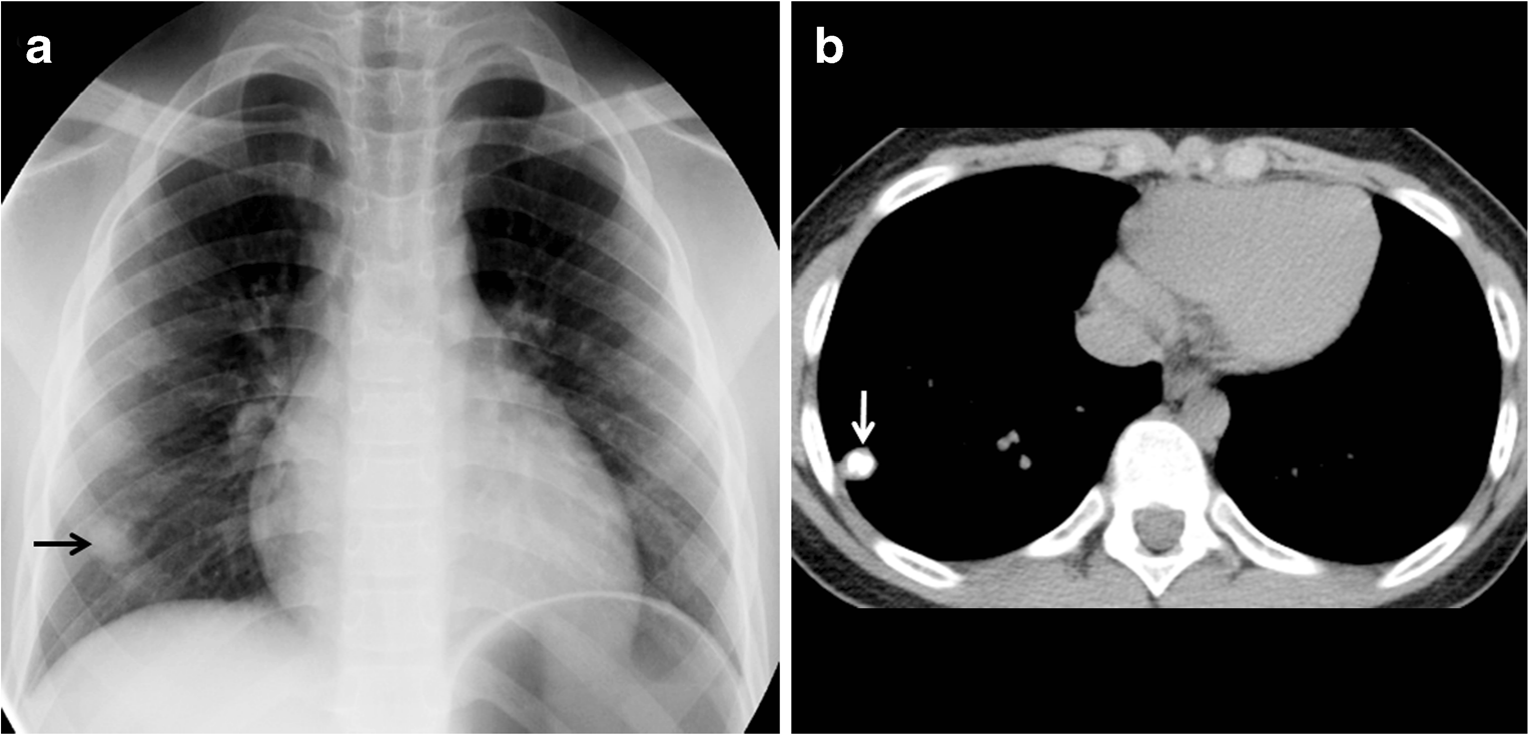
Ghon focus in a 7-year-old boy. a Anteroposterior chest radiograph shows a Ghon focus (*arrow*) in the right lower lobe. b Non-enhanced axial chest CT demonstrates the focus, which is calcified (*arrow*)
